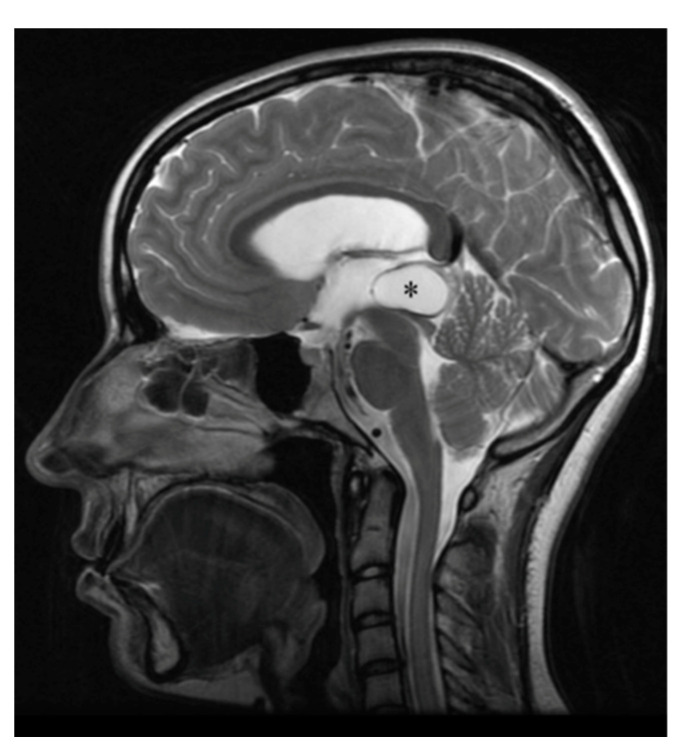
Pineal cyst MRI. The asterisk indicates the pineal cyst. Reprinted with permission from Májovský et al. (2017) [124]—Elsevier—2021 (License Number 5020240575766).
